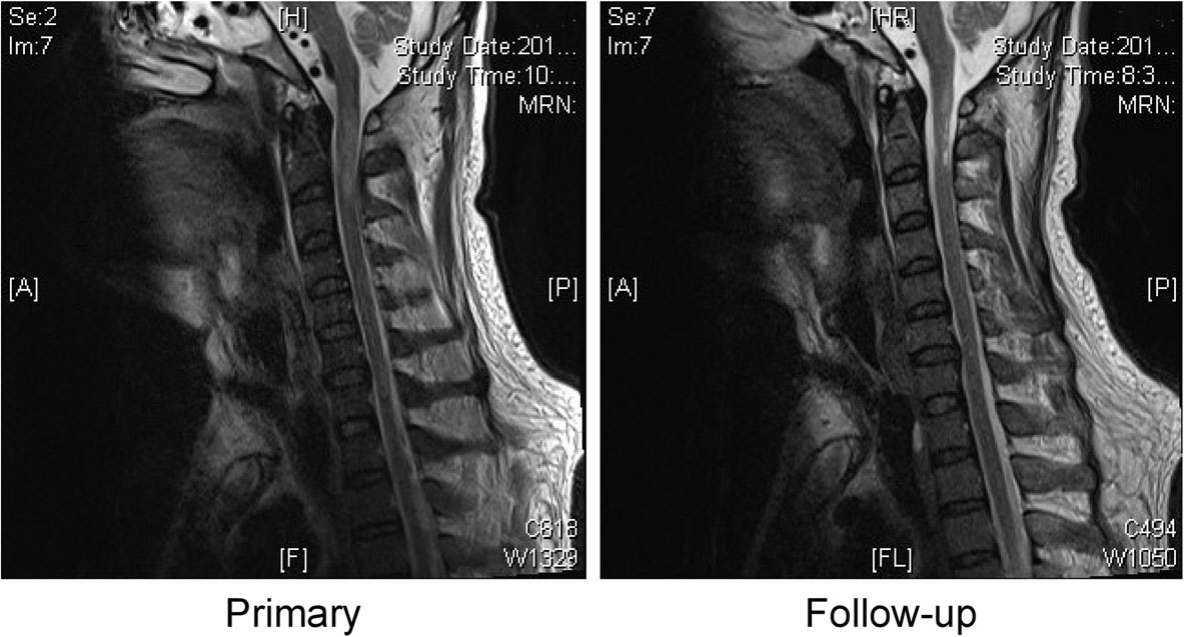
A follow-up cervical MRI evaluation revealed a less extensive hyperintense region on sagittal sections of T2-weighted images
